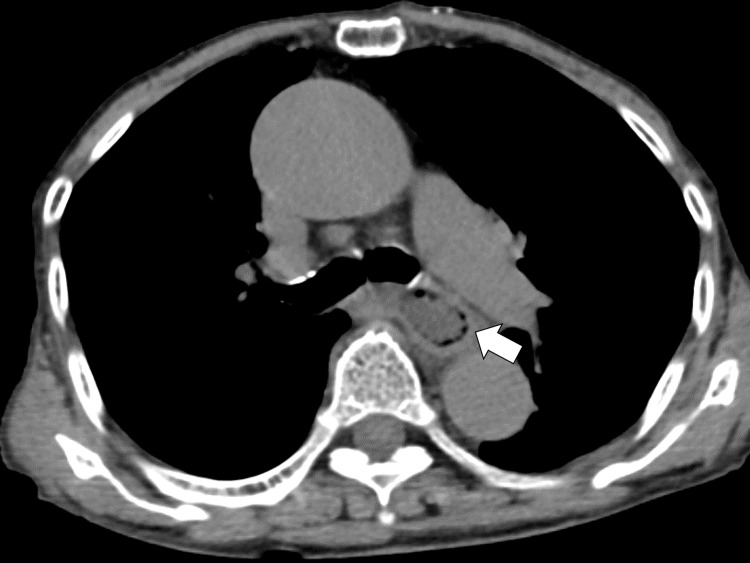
Computed tomography of the chest shows gas-accumulated lesions on the wall of the esophagus.
